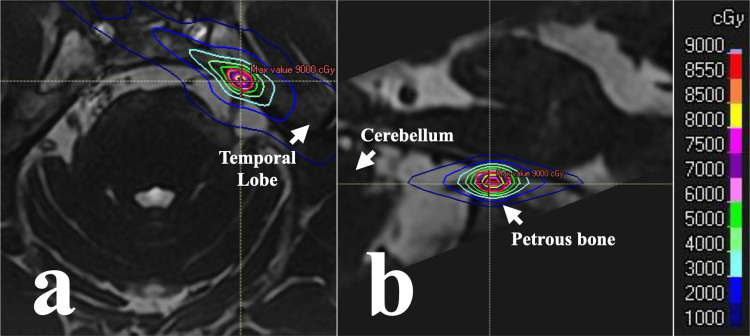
Dose distribution of SRS (Case 1). Isodose lines are shown relative to the maximum dose (100% = 90 Gy) on high-resolution T2WI. (a) Axial view and (b) sagittal view. SRS: stereotactic radiosurgery; T2WI: T2-weighted imaging.
